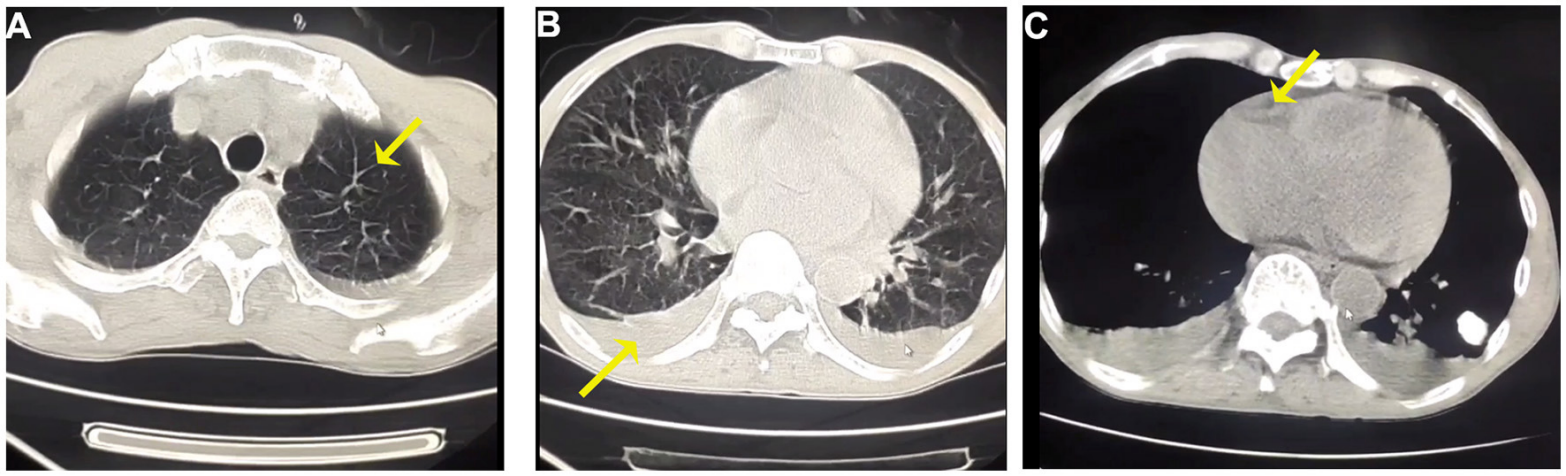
(A) Representative CT images of interlobular septal thickening at chest CT scan of both upper lungs. (B) Bilateral pleural effusions in the lower lungs. (C) The mediastinal window of the chest CT reveals a small amount of pericardial effusion.
